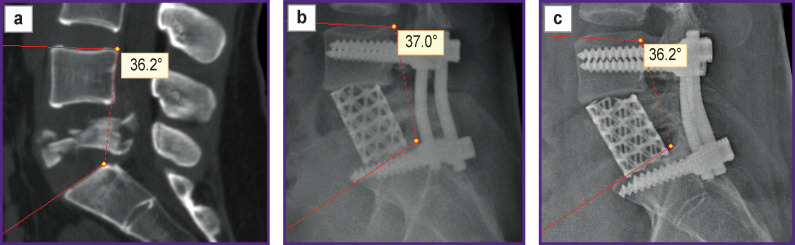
CT scan and X-ray examination of patient P., 29 years old: (a) 7 days after injury; (b) 7 days after transpedicular fixation and anterior column support with a Mesh; (c) 12 months after transpedicular fixation and anterior column support with a Mesh
